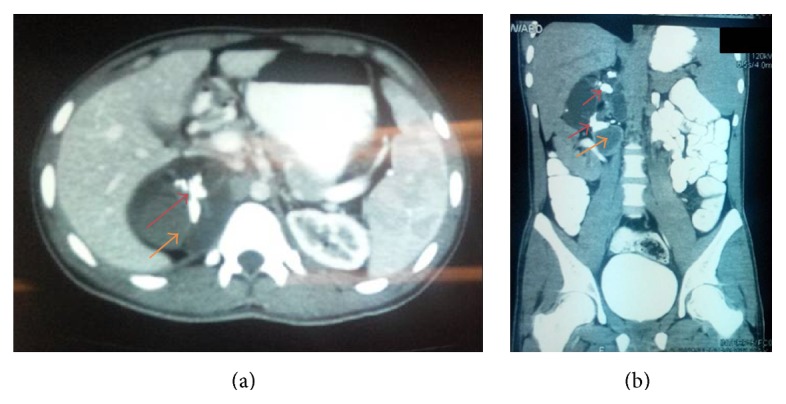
Contrast enhanced CT scan showing cystic mass with solid component (marked with orange arrow) and areas of calcification (marked with red arrow). (a) Axial section. (b) Coronal section.
